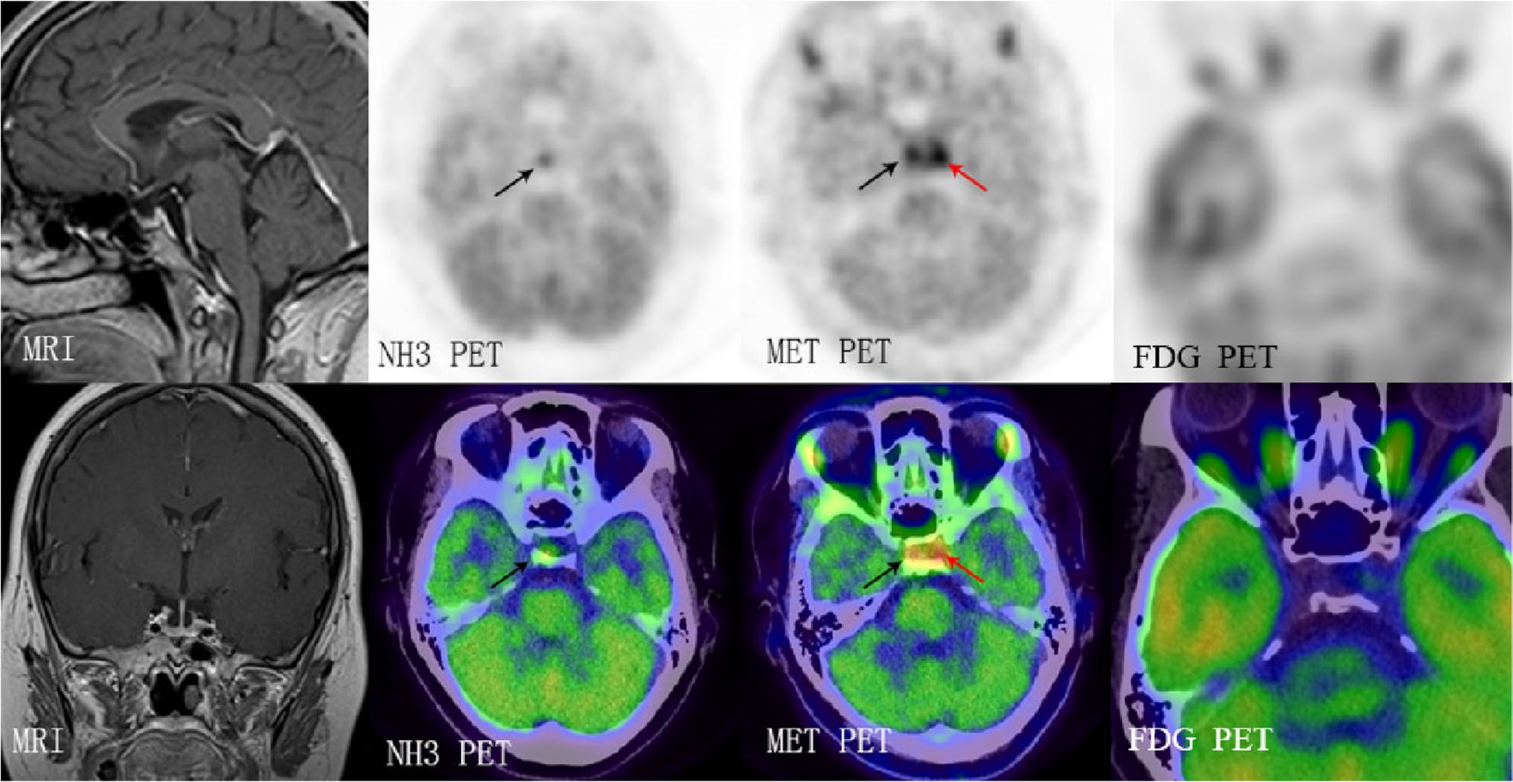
Overlap of increased [^11^C]C-MET uptake in tumors and pituitary tissue. [^11^C]C-MET PET/CT images showed that both tumors and pituitary tissue had a high [^11^C]C-MET uptake, while only pituitary tissue had a high [^13^N]N-NH3 uptake. Neither tumors nor pituitary tissue was detected by [^18^F]F-FDG PET
